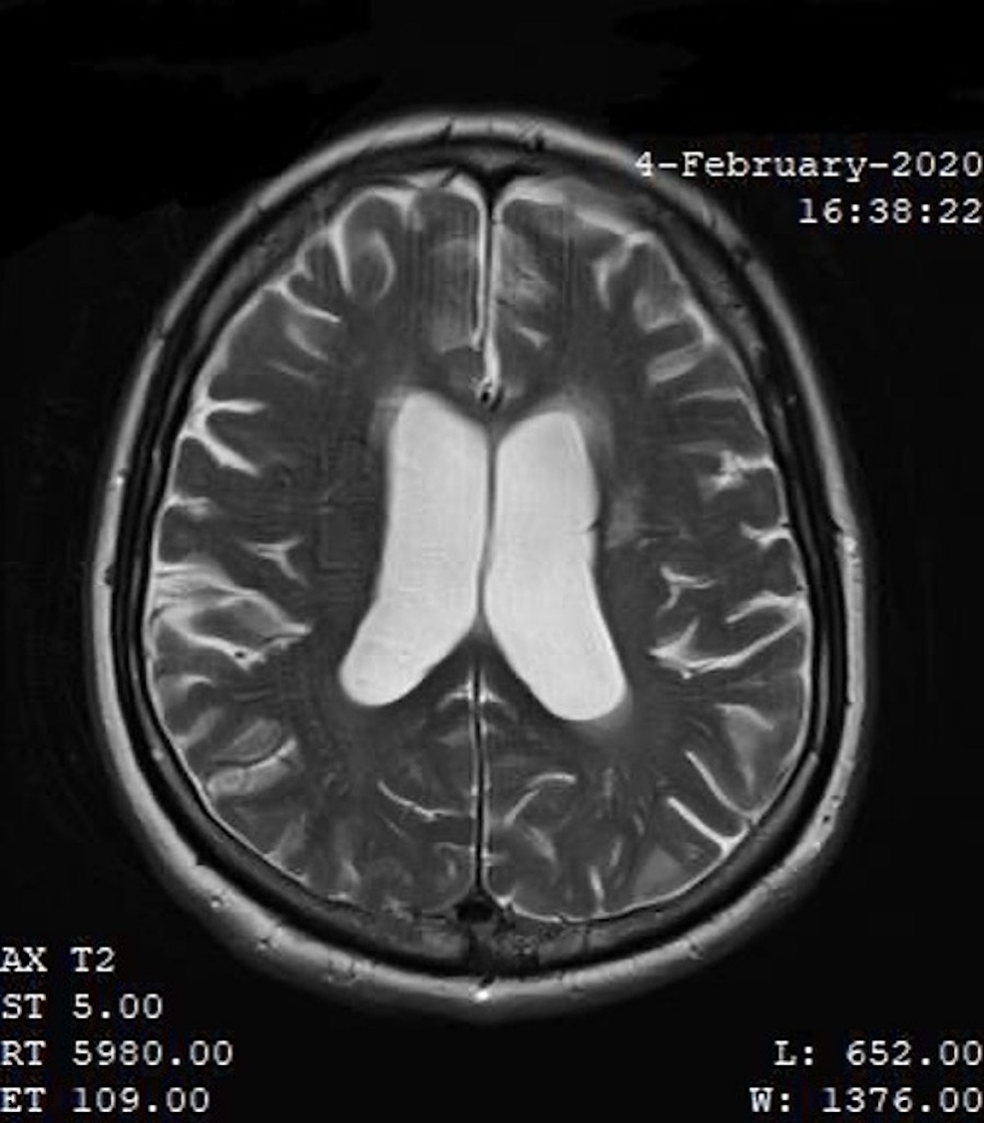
Patient MRI performed on February 4, 2020
